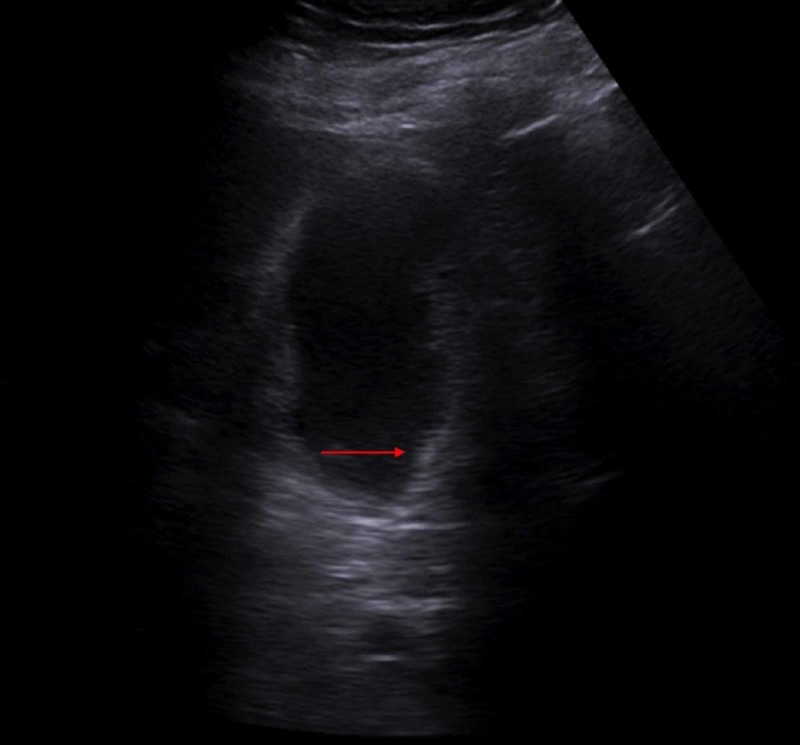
Ultrasound showing inspissated gall bladder (arrow pointing)
